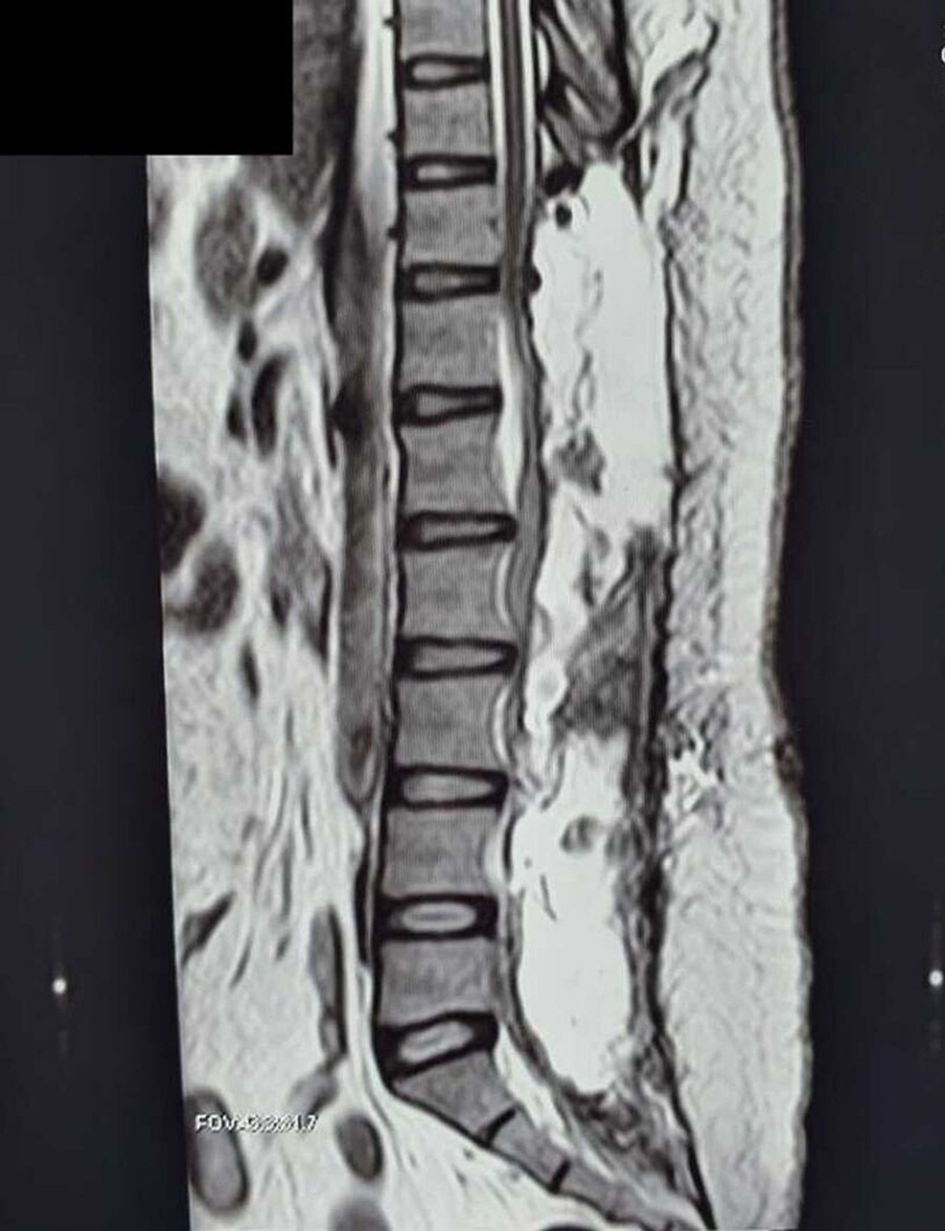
Sagittal T2-weighted image of spine showing removal of the tumor
